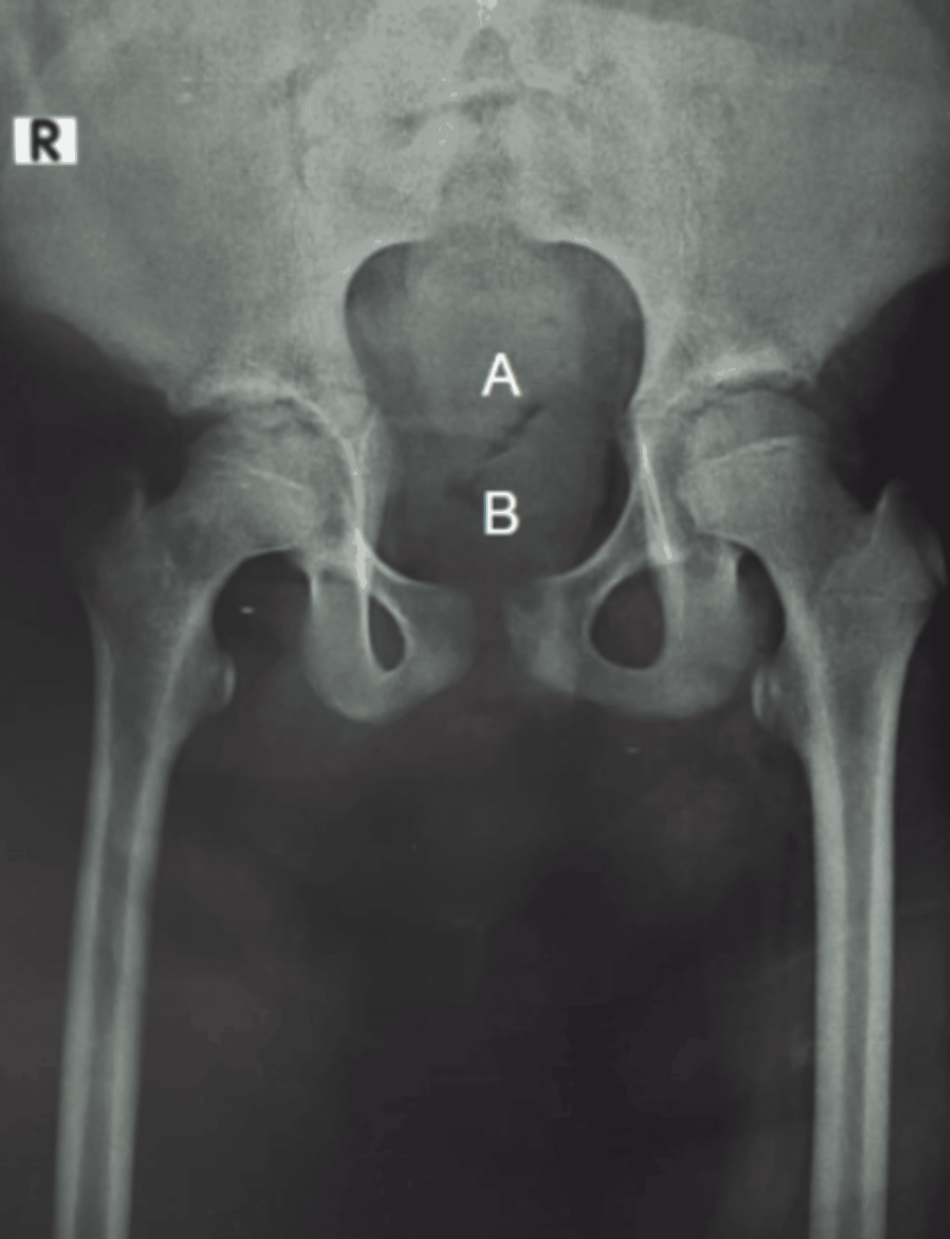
Frontal radiograph of the pelvis showing non-visualization of the (A) sacrum and (B) coccyx.
